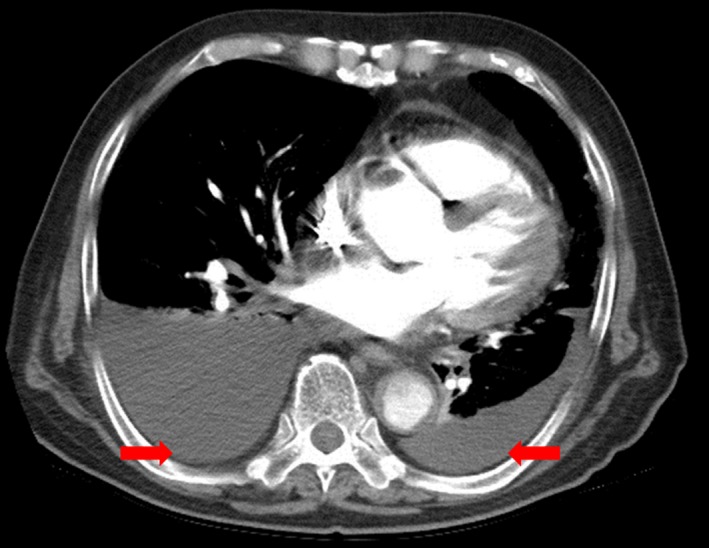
Chest computed tomography on admission showing bilateral pleural effusions (arrows) without any swelling of the lymph nodes
